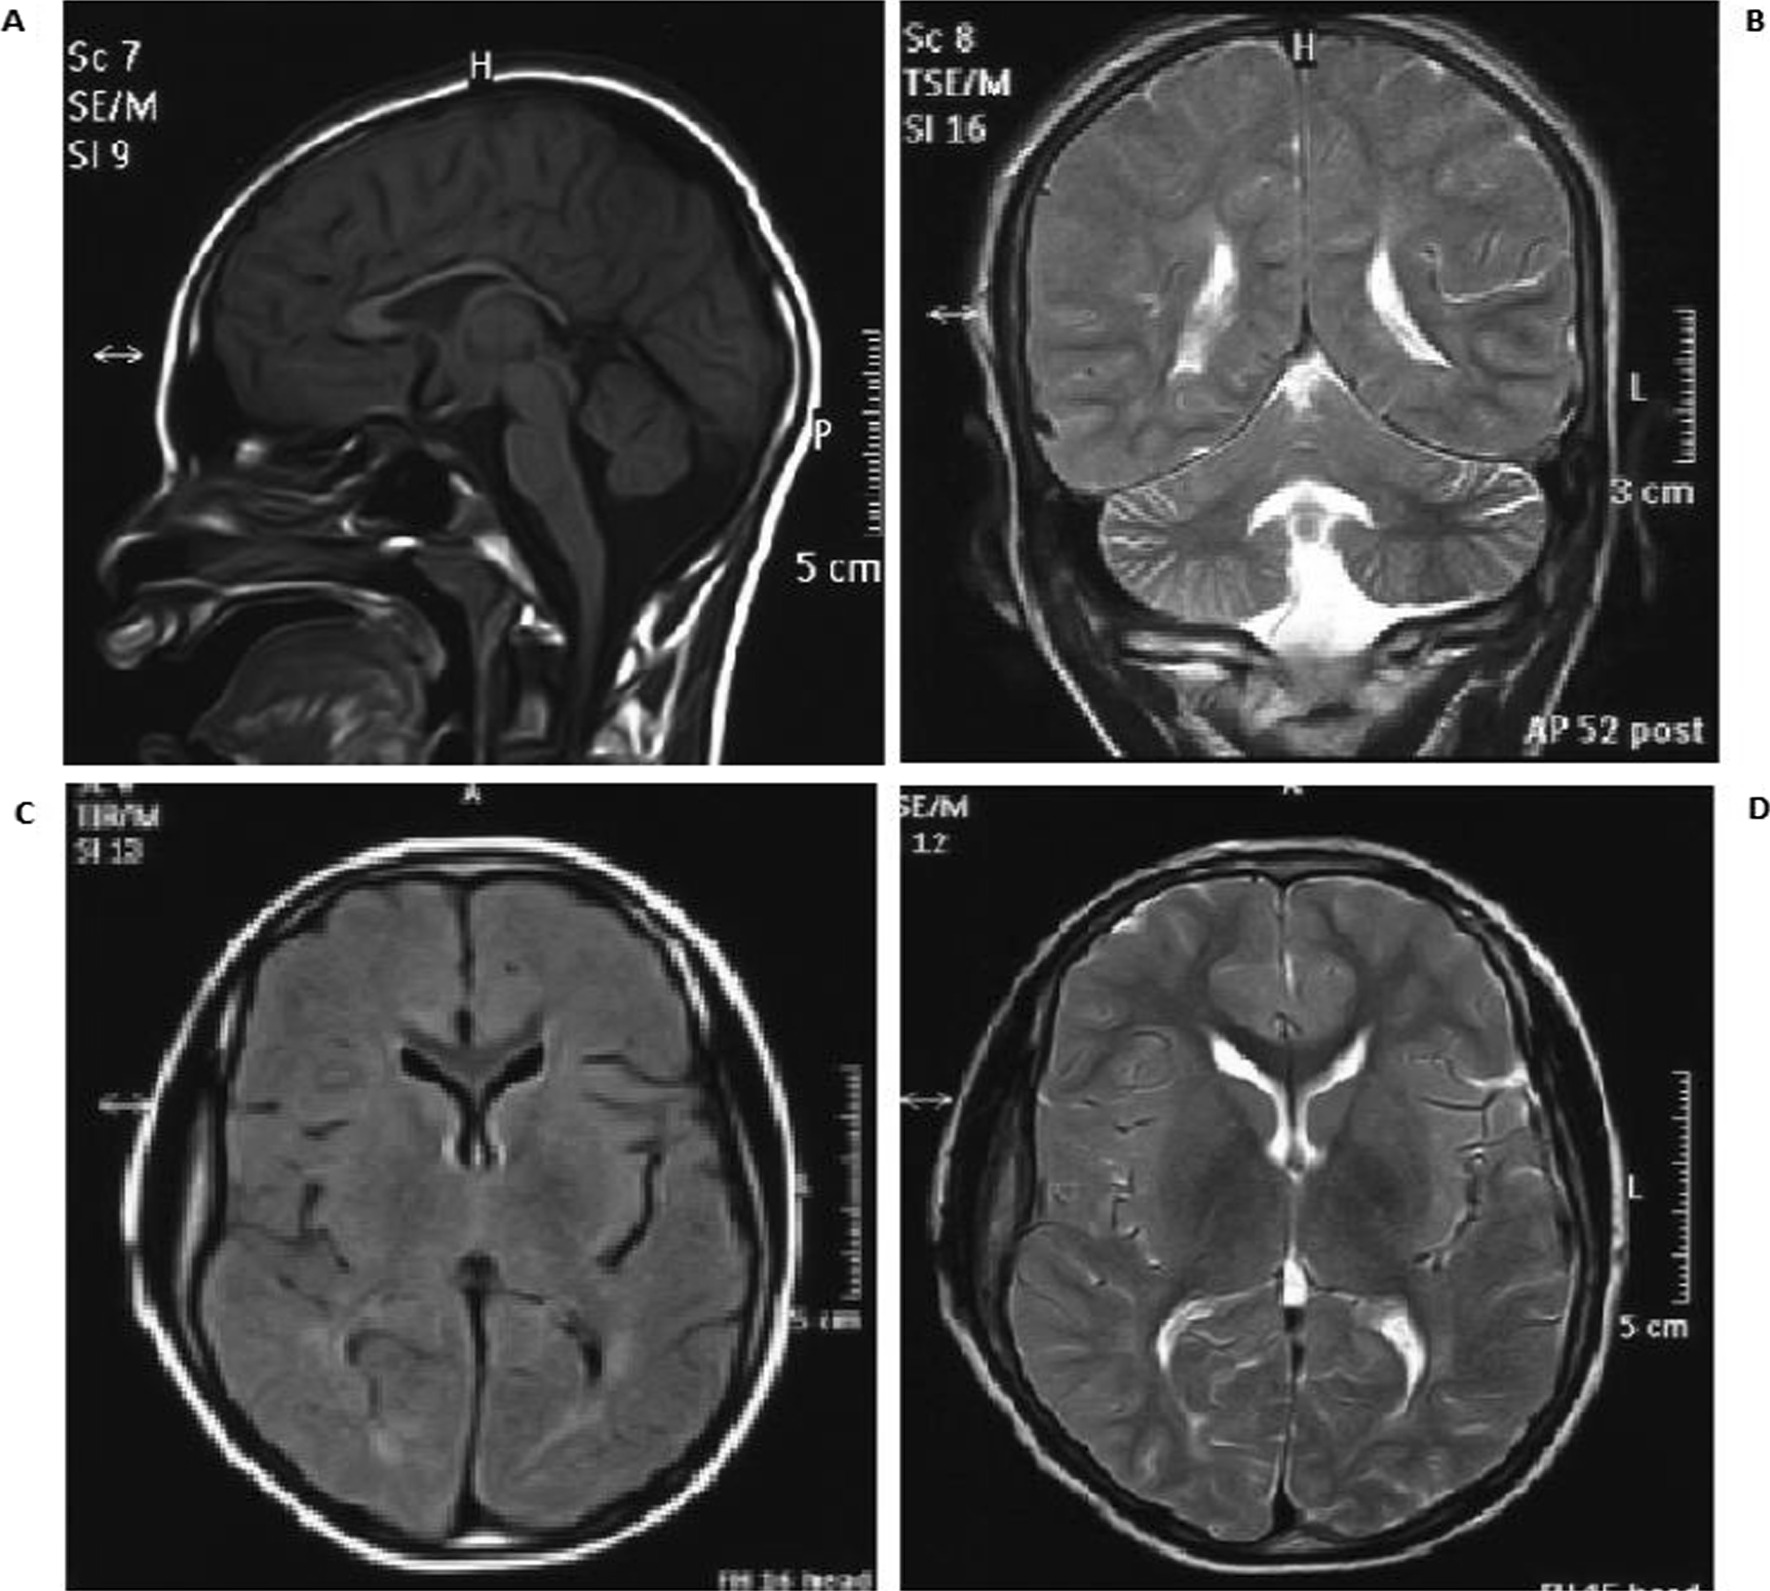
Brain MRI (T1) of patient one showing mild cortical and cerebellar atrophy, white matter abnormality and thin corpus callosum (A: T1 (sagittal section), B: T2 (coronal section), C: T1 (cross section), D: T2 (cross section))
